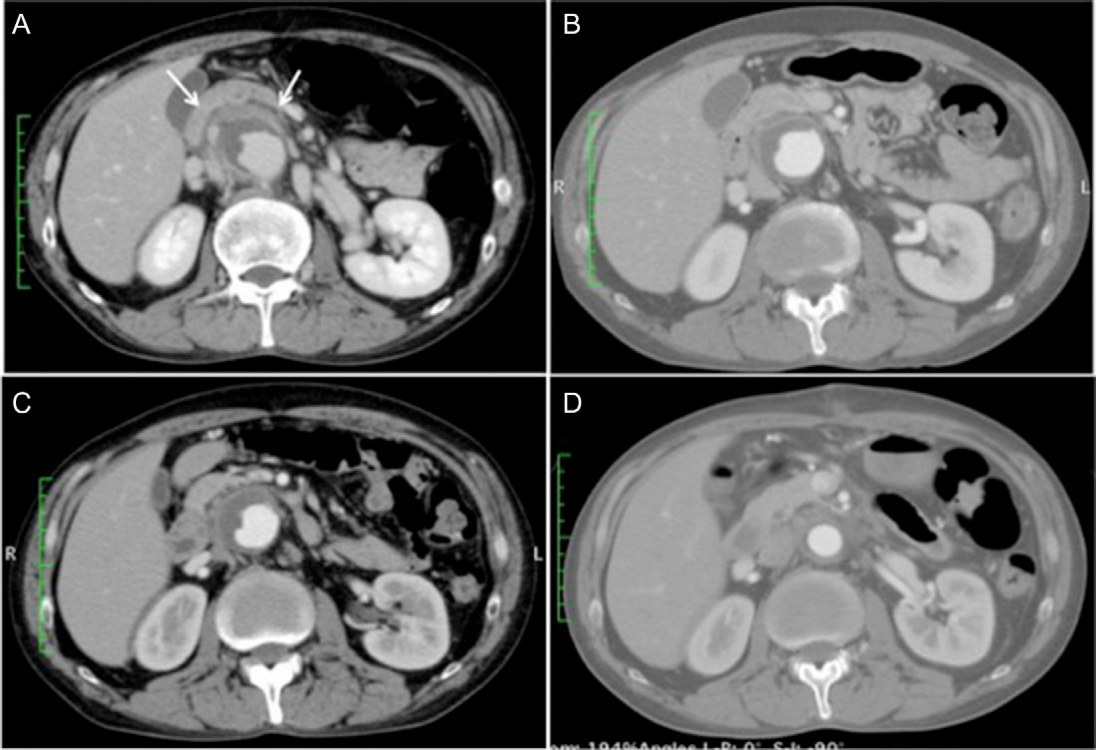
(A) Contrast-enhanced computed tomography scan at the time of admission showing a 50-mm juxtarenal abdominal aortic aneurysm surrounded by dense fibrous tissue (white arrows). (B and C) The perianeurysmal fibrous tissue became smaller along with the course of steroid therapy, 7 and 14 days after the initiation of steroid therapy, respectively. (D) Postoperative computed tomography scan showing a patent graft with near disappearance of the fibrous tissue.
